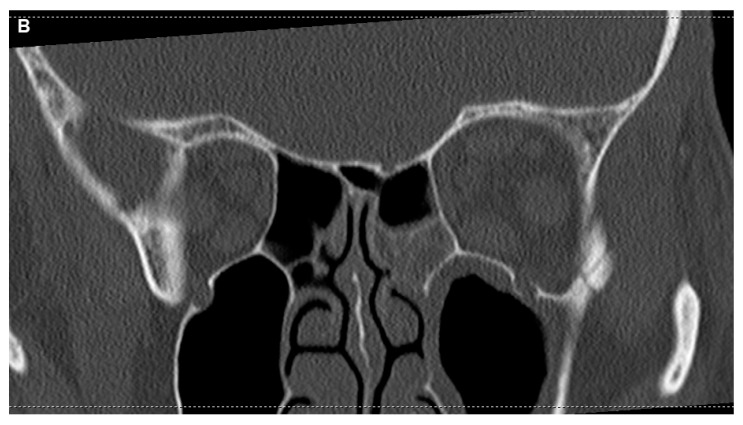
CT imaging of the orbit and paranasal sinuses. The axial view reveals soft tissue swelling of the left periorbital region, with both globes appearing intact. There was no evidence of intra- or extraconal hematoma or abscess. Notably, increased vascular markings and fat imbibition within the left orbit as well as swelling of the external orbital muscles were visible, suggesting inflammatory changes. Mucosal swelling was present in the paranasal sinuses; however, the bone structures were not affected at all (A). A coronal view shows partial opacificagion of the left sphenoid sinus, which retinas some aeration. Despide this, following further consultation with ENT specialists, it was considered unlikely that the paranasal sinuses were the primary source of the patient’s symptoms. While partial opacification of the left sphenoid sinus was observed, it remained partially aerated.
